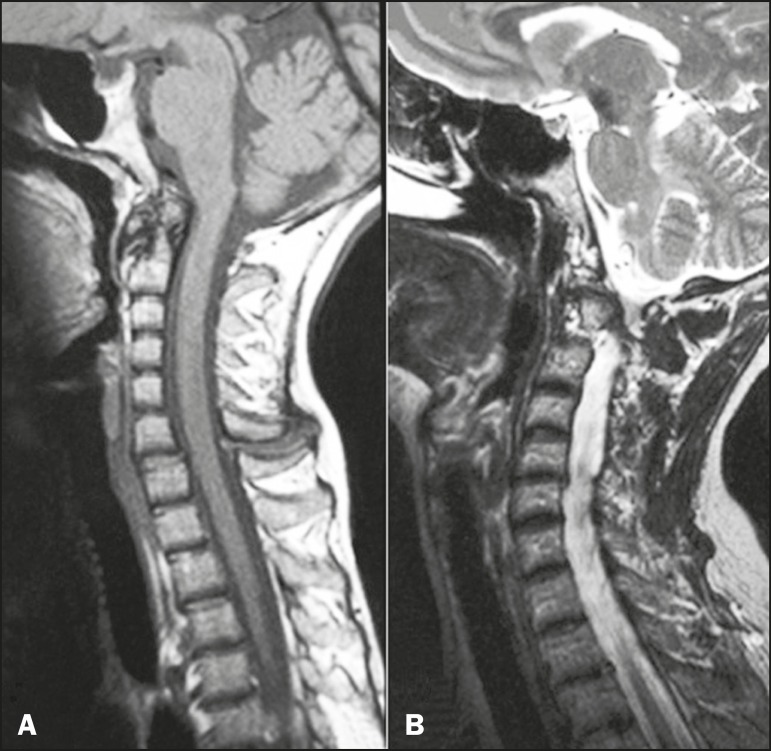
A female patient diagnosed with rheumatoid arthritis. T1-weighted and T-2
